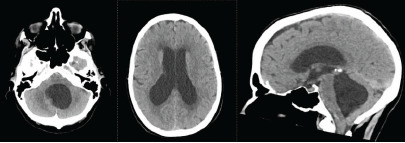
Head computed tomography on admission demonstrating hydrocephalus with enlargement of the lateral ventricles, 3rd ventricle, and 4th ventricle, with downward descent of the cerebellar tonsils.
